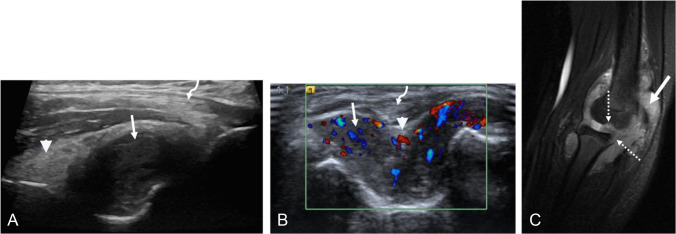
An 8-year-old girl with a known diagnosis of juvenile idiopathic arthritis presenting with elbow swelling. Long-axis (A) and axial (B) ultrasound images of the posterior elbow demonstrate the U-shaped concavity of the olecranon fossa filled with a hypoechoic, hyperemic region of synovitis (*arrow*). The posterior fat pad is elevated (*arrowhead*). The triceps tendon (*curved arrow*) appears echogenic and fibrillar, seen superficial to the posterior joint recess as it extends toward its insertion on the olecranon. C MR sagittal T2 fat saturation sequence in the same patient demonstrating the extent of severe synovitis (*arrow*) and erosive changes (*dashed arrows*) at the radiocapitellar articulation
